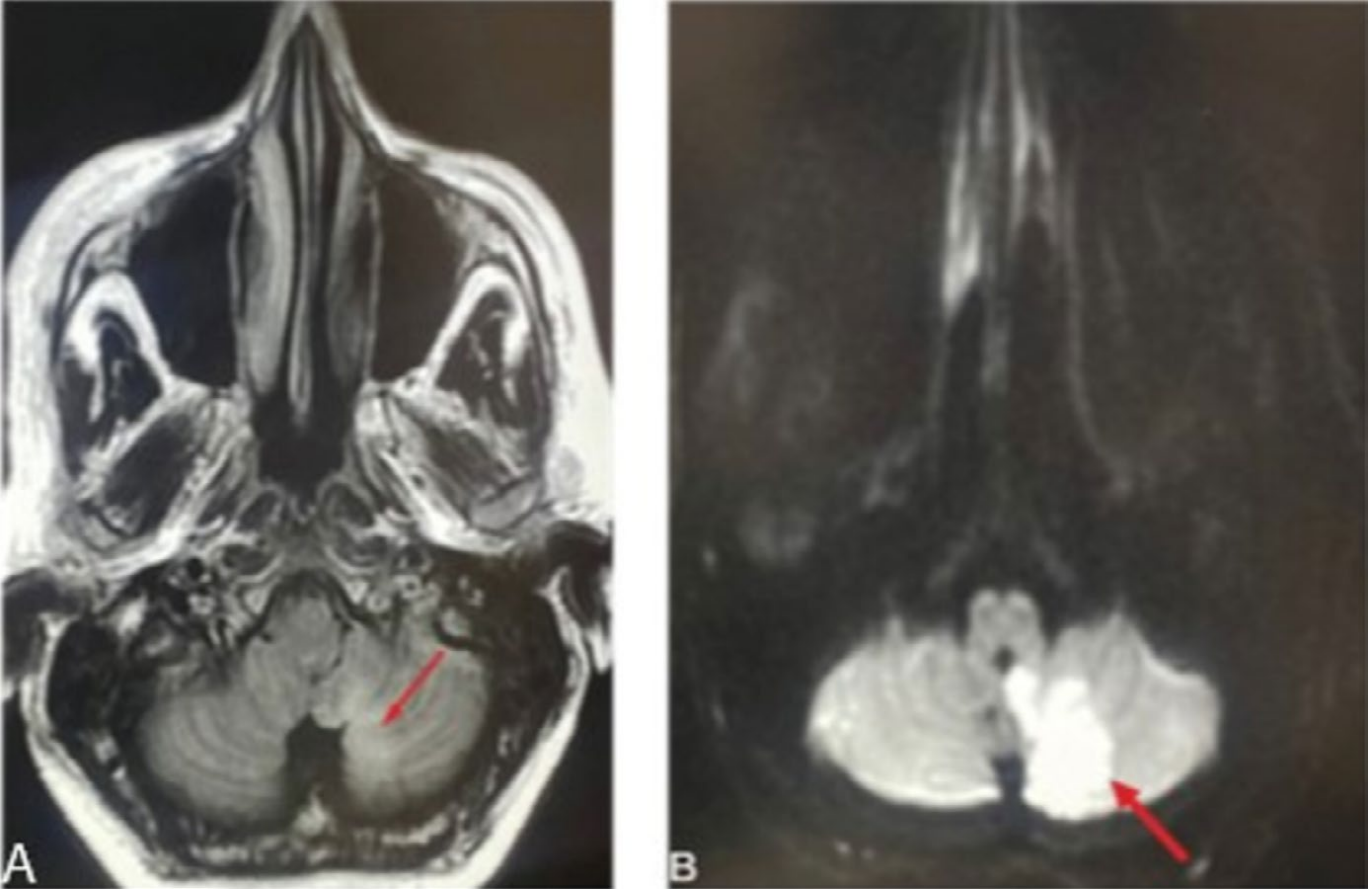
(A) Shows a T1 intensity MRI, and the arrow points hypointensity in the left cerebellar hemisphere, in the inferior medial portion. (B) shows diffusion restriction MRI, and the arrow points to the area of the diffusion restriction. These changes represent acute ischemic infarction in the left posterior inferior cerebellar artery (PICA) territory without hemorrhagic transformation.
